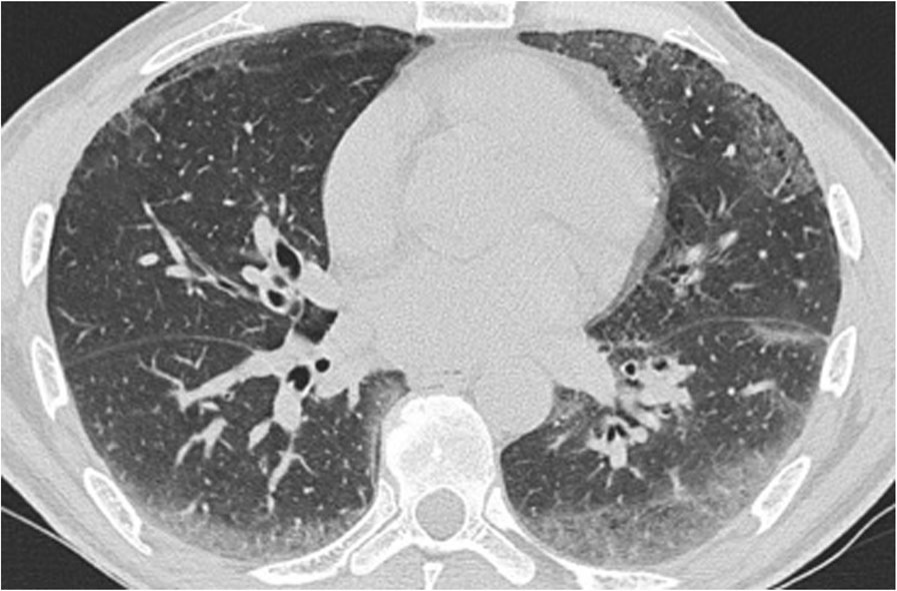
Chest HRCT showing a CT pattern indeterminate for UIP (fibrosis with ground-glass suggestive of non-UIP pattern)
